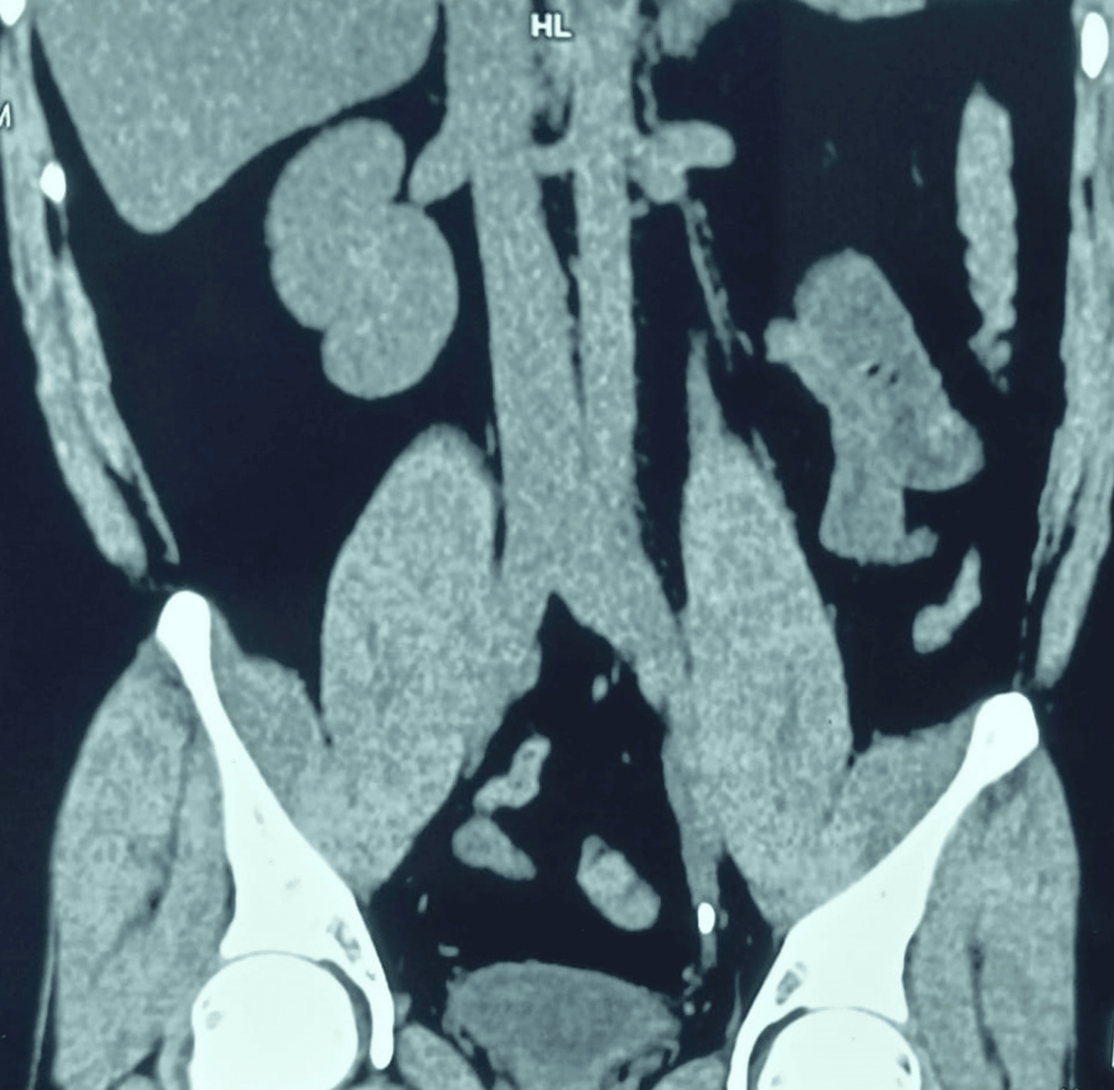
Non-contrast CT image showing 8.5x3.5 mm left distal ureteric calculus with proximal hydroureteronephrosis CT: computed tomography
